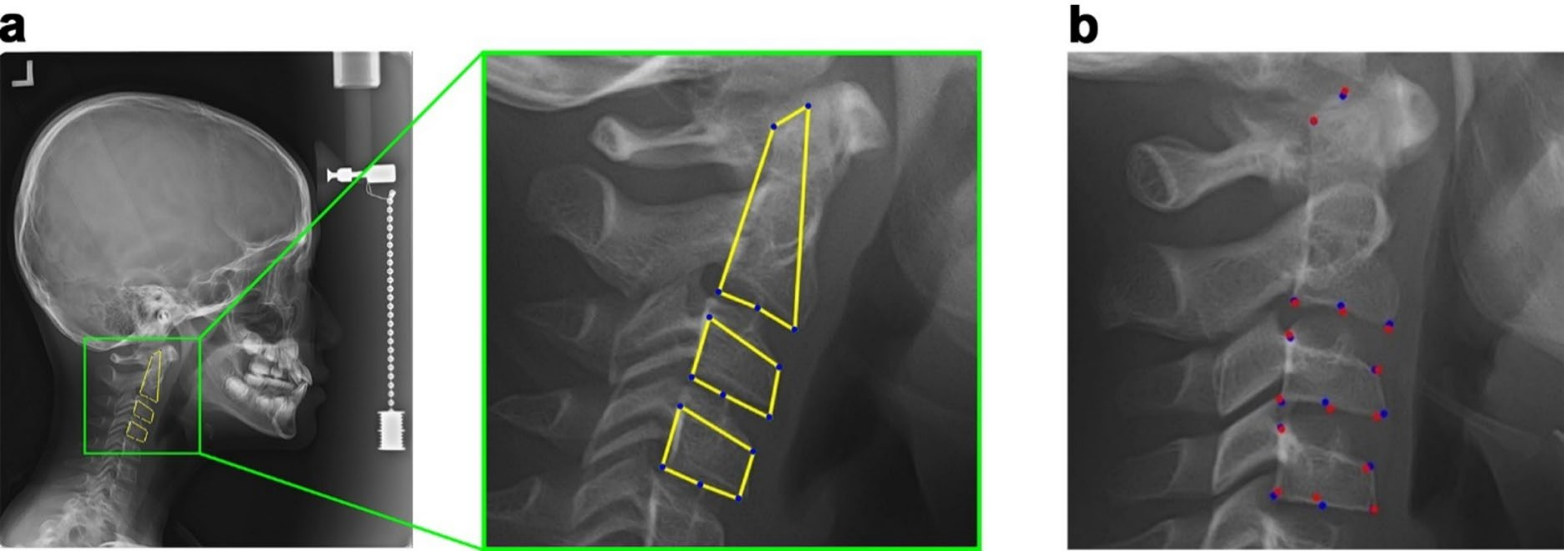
Automated region of interest (ROI) detection and landmark detection on lateral cephalometric radiograph. (a) Original lateral cephalometric radiograph with the detected C2–C4 ROI indicated by a yellow-green bounding box. The isolated and resized (224 × 224 pixels) ROI is shown alongside. The square format preserves vertebral aspect ratios critical for accurate CVM classification. (b) Coordinates for the 15 anatomical points on C2-C4 vertebrae are shown in red (ground-truth) and blue (predicted).
